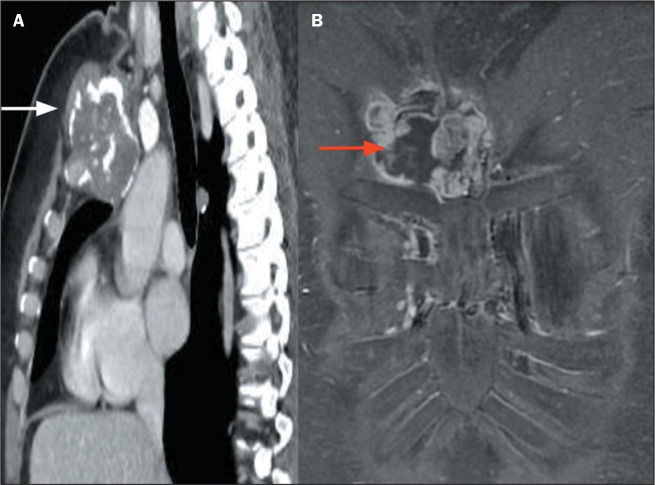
Chondrosarcoma. A: Reconstruction of a contrast-enhanced sagittal CT scan
